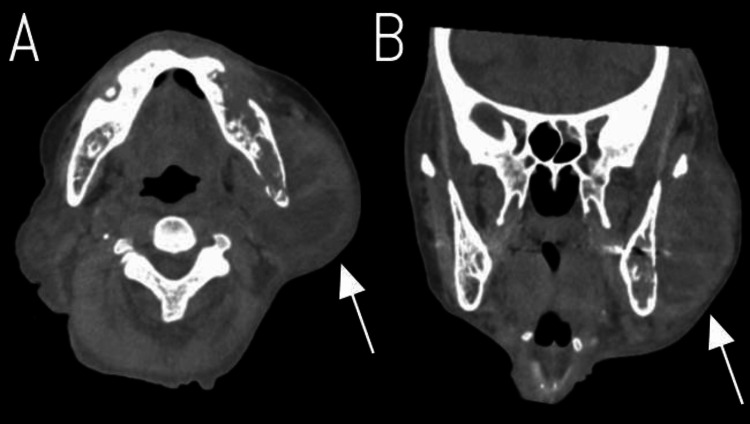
Head computed tomography on hospital day 7 revealing a left perimandibular abscess (A) Axial view and (B) coronal view demonstrating a large, well-defined fluid collection in the left perimandibular region (white arrows). The abscess shows characteristic rim enhancement and causes mass effect with compression of surrounding structures.
